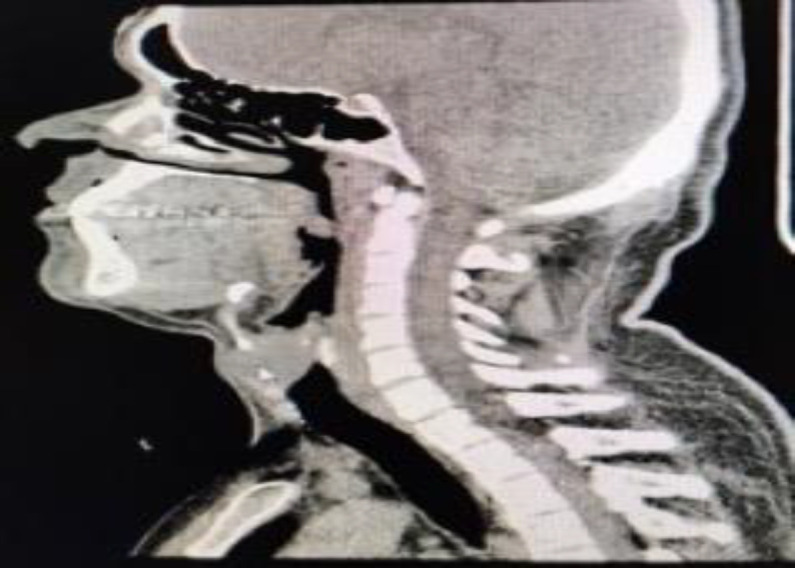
Computed tomography showing the cyst between the thyroid and cricoid cartilage with projection into the airway lumen
